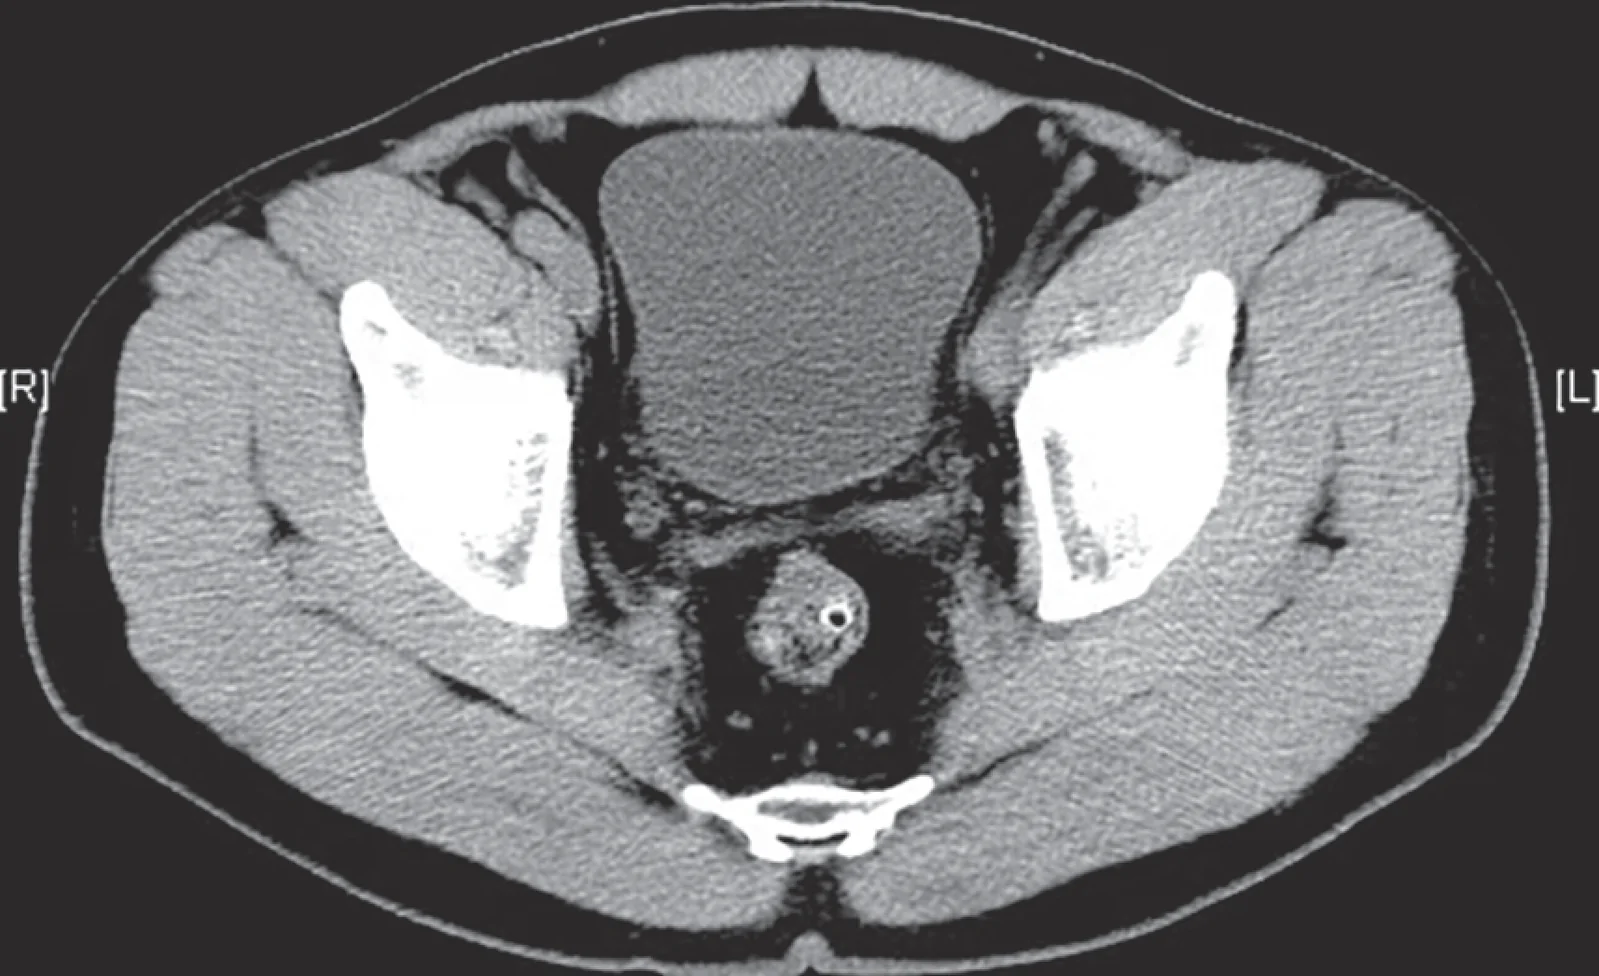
A CT scan revealed a well-defined, round/ovoid, focally
